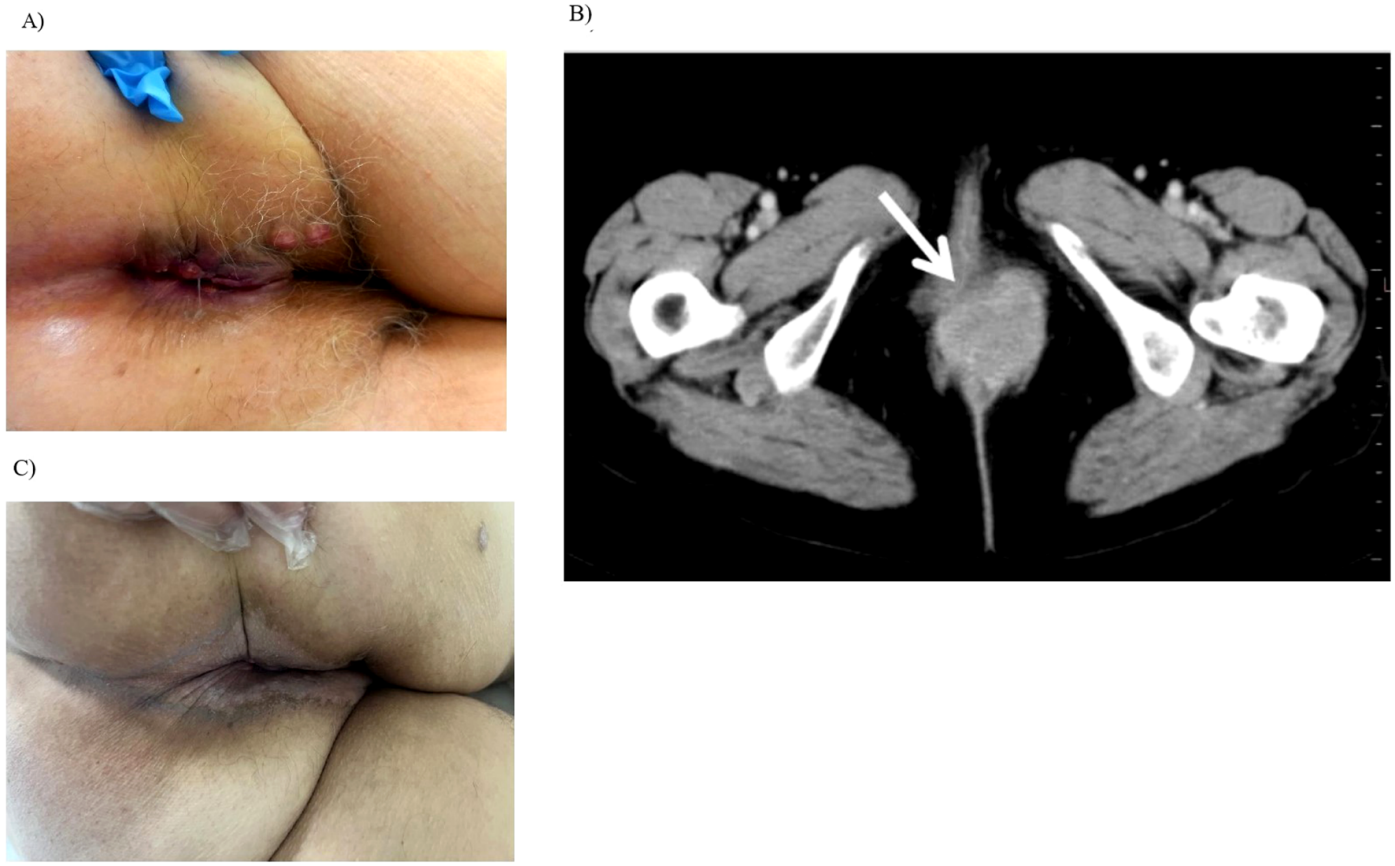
Clinical and radiological findings: Local effects before and after CRT (A) Clinical examination showing an extensive anal canal tumor with contiguous spread to the labia majora and adjacent perianal skin. (B) Axial CT scan demonstrating a bulky anal canal mass with proximal extension into the rectum and anterior infiltration of the labia majora. (C) Clinical presentation after CRT showing complete local response. The previously extensive lesion has fully resolved, with no visible or palpable signs of disease.
